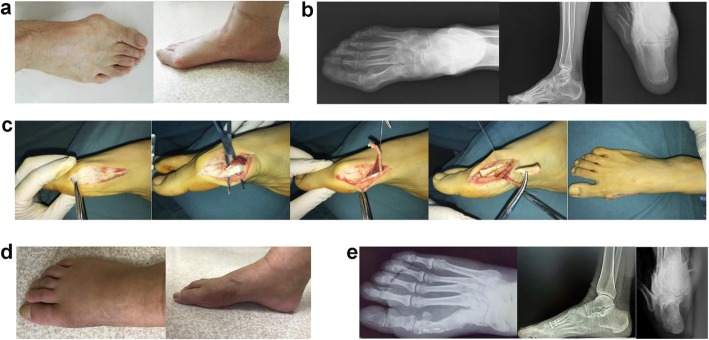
A 69–year-old woman had right-sided hallux valgus deformity. a to b Digital photos and X-ray showing the hallux valgus deformity. c The process of surgical technique. d Digital photos and X-ray after 24-month follow up
